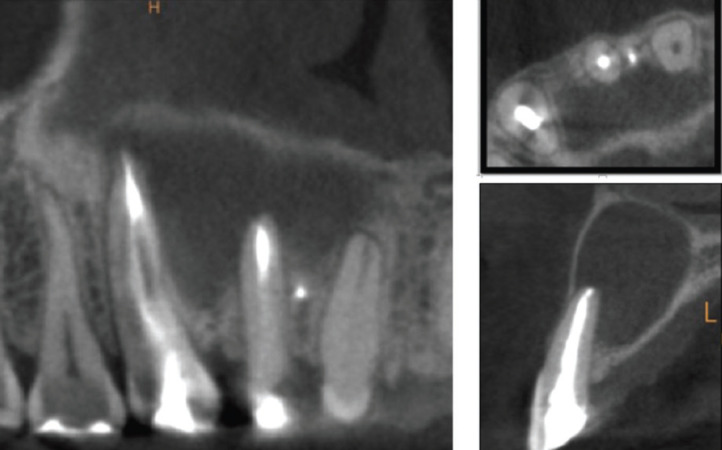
CBCT Coronal, axial and sagittal views of superior right lateral incisor with accessory root presenting a big periapical radiolucency.
